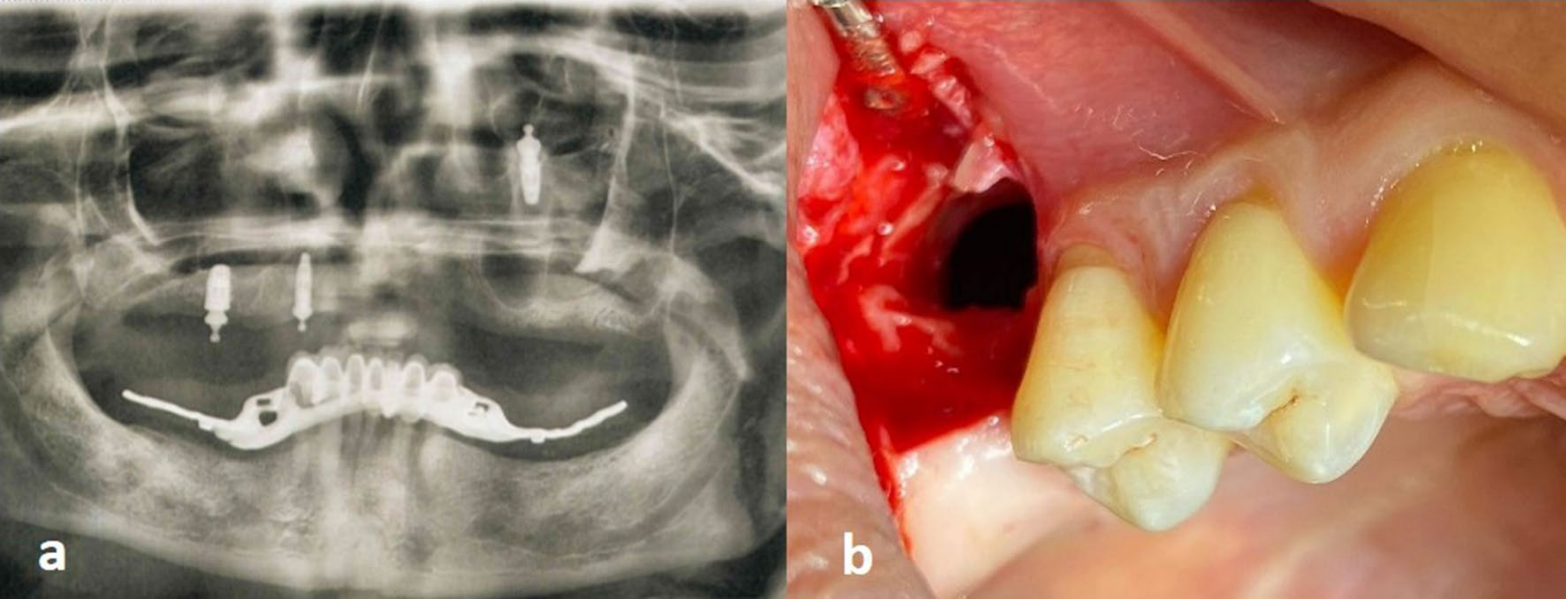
An X-ray showing a dental implant with ball abutment displaced toward the superior part of the maxillary sinus 6 months after function (a); upper lateral surgical approach to access displaced implant in the superior part of the sinus using two sided flap (b).
